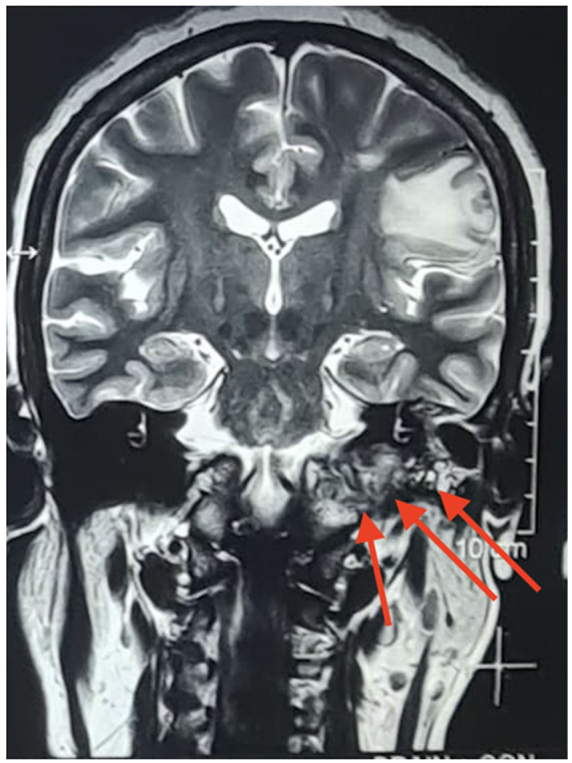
Coronal T2-weighted MRI image showing a soft tissue lesion at the base of the skull on the left side (indicated by red arrows). The lesion appears iso-intense on T2W and causes lytic destruction of the left mastoid air cells, with extension into the middle ear cavity.
